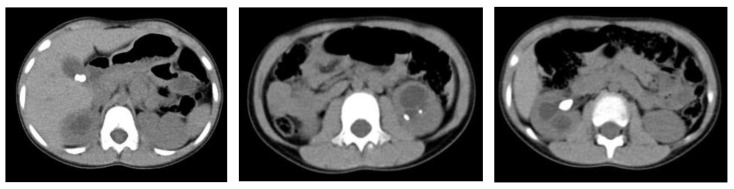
Abdominal computed tomography shows a gallstone and renal stones.
